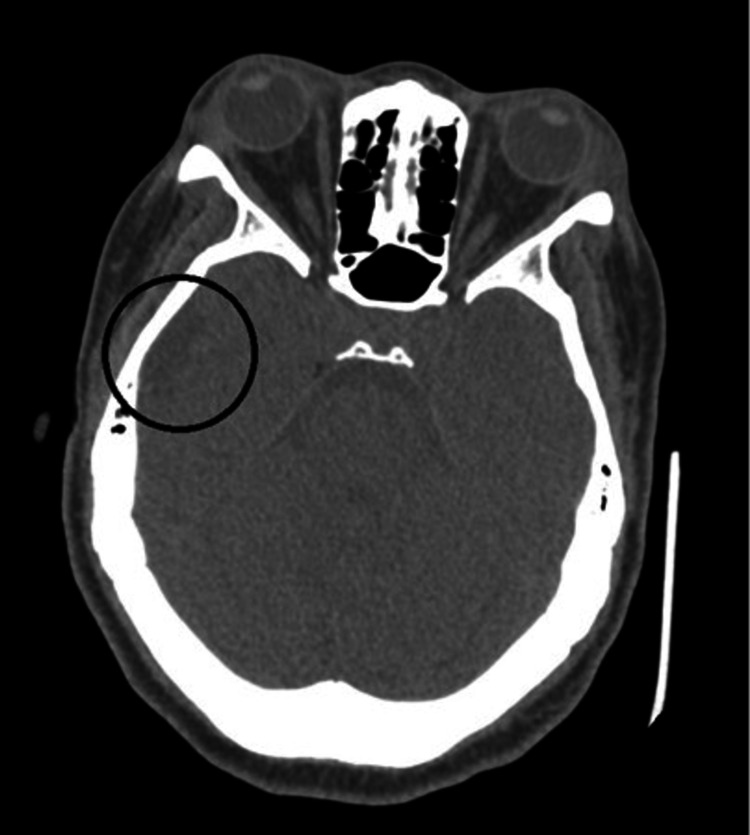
Computed tomography head showing lateral right temporal encephalomalacia
